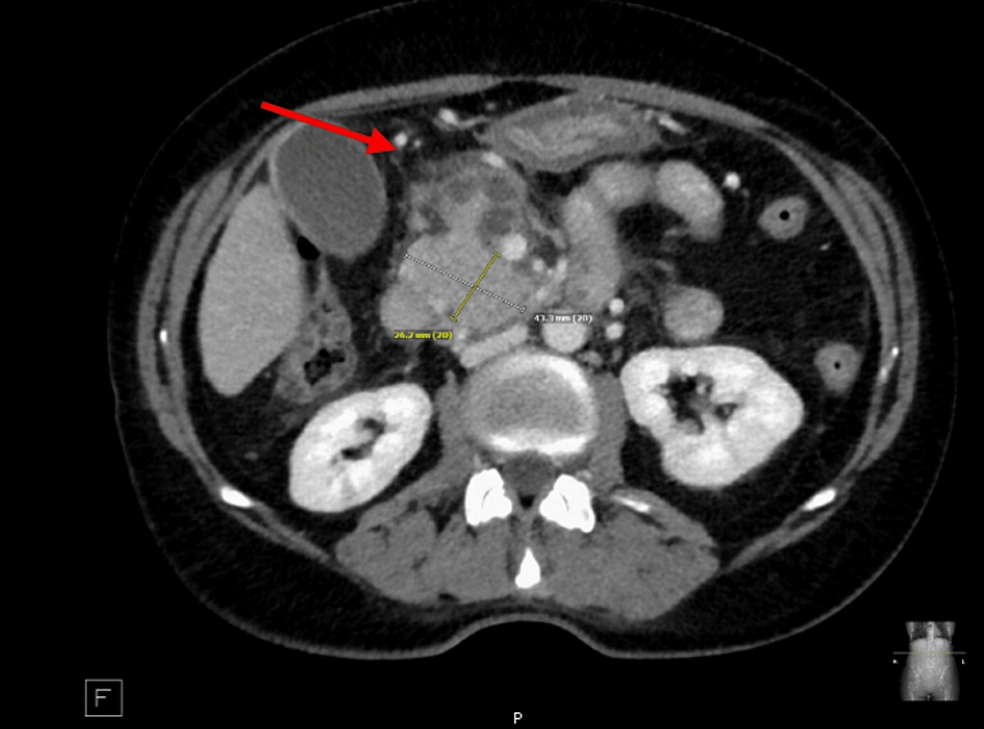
CT abdomen/pelvis showing a 4.3 cm pancreatic head mass
